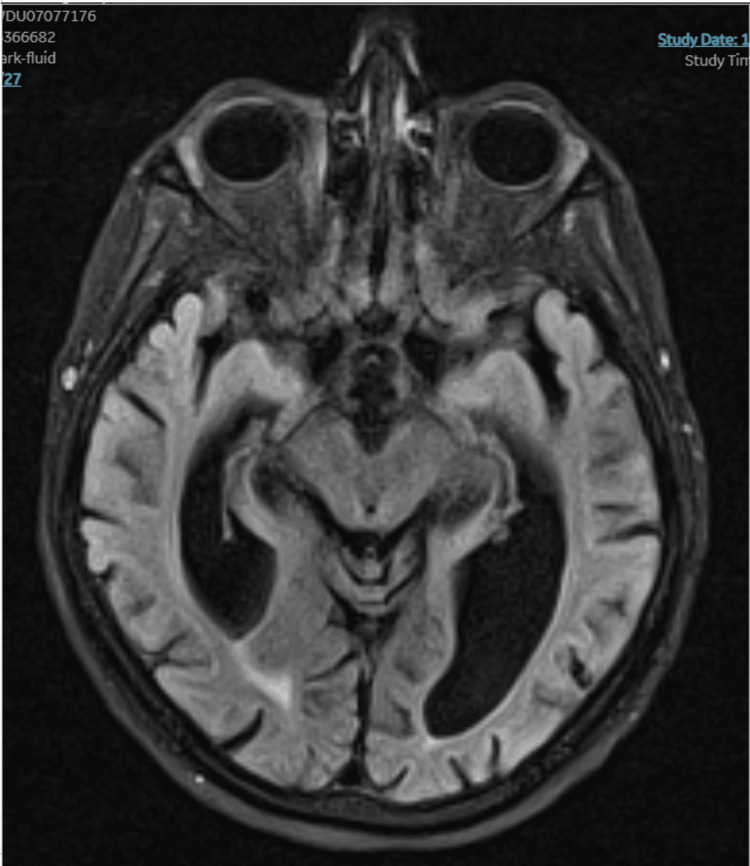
T2 FLAIR hyperintensities in the mammillary bodies and periaqueductal gray matter Axial T2-weighted FLAIR magnetic resonance imaging of the brain demonstrates symmetrical hyperintense signal abnormalities involving the medial thalami, mammillary bodies, and periaqueductal gray matter, which are characteristic regions affected in Wernicke's encephalopathy. These findings support the clinical diagnosis in the context of thiamine deficiency risk factors and compatible neurological symptoms. The imaging was obtained during the patient's acute hospitalization. No contrast enhancement was required for diagnostic interpretation. FLAIR: fluid-attenuated inversion recovery
